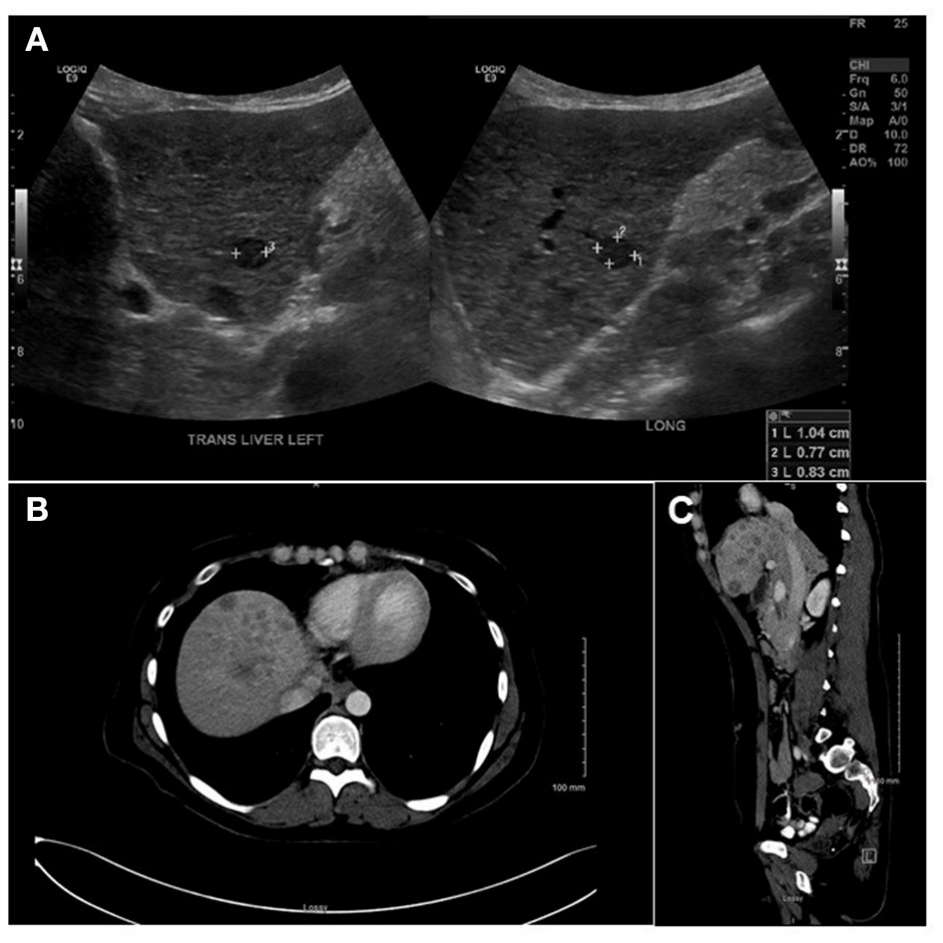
(A) Liver ultrasound showing multiple hypo-echoic lesions ranging between 0.5 and 3.3 cm. (B) A liver computerized tomography scan showing multiple hypo-echoic lesions with peripheral rim of increased enhancement. (C) A magnetic resonance imaging (sagittal view) showing same as (A,B).
